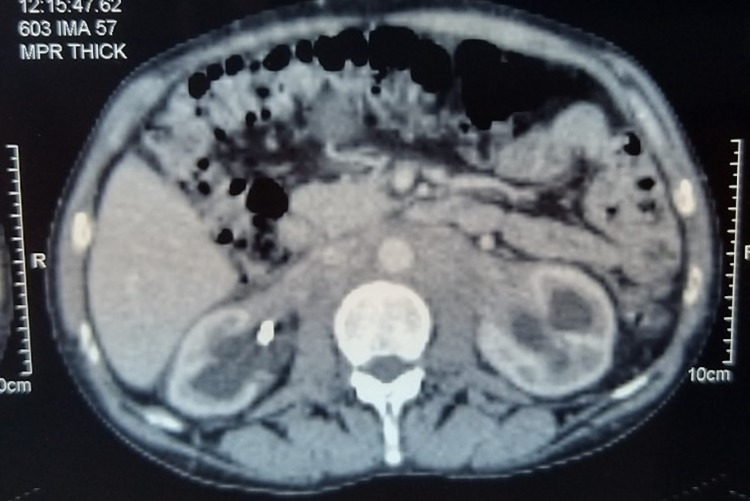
Abdominal CT scan, after contrast product injection, showing retroperitoneal fibrosis with a perivascular fibrous sheath and bilateral hydronephrosis.
